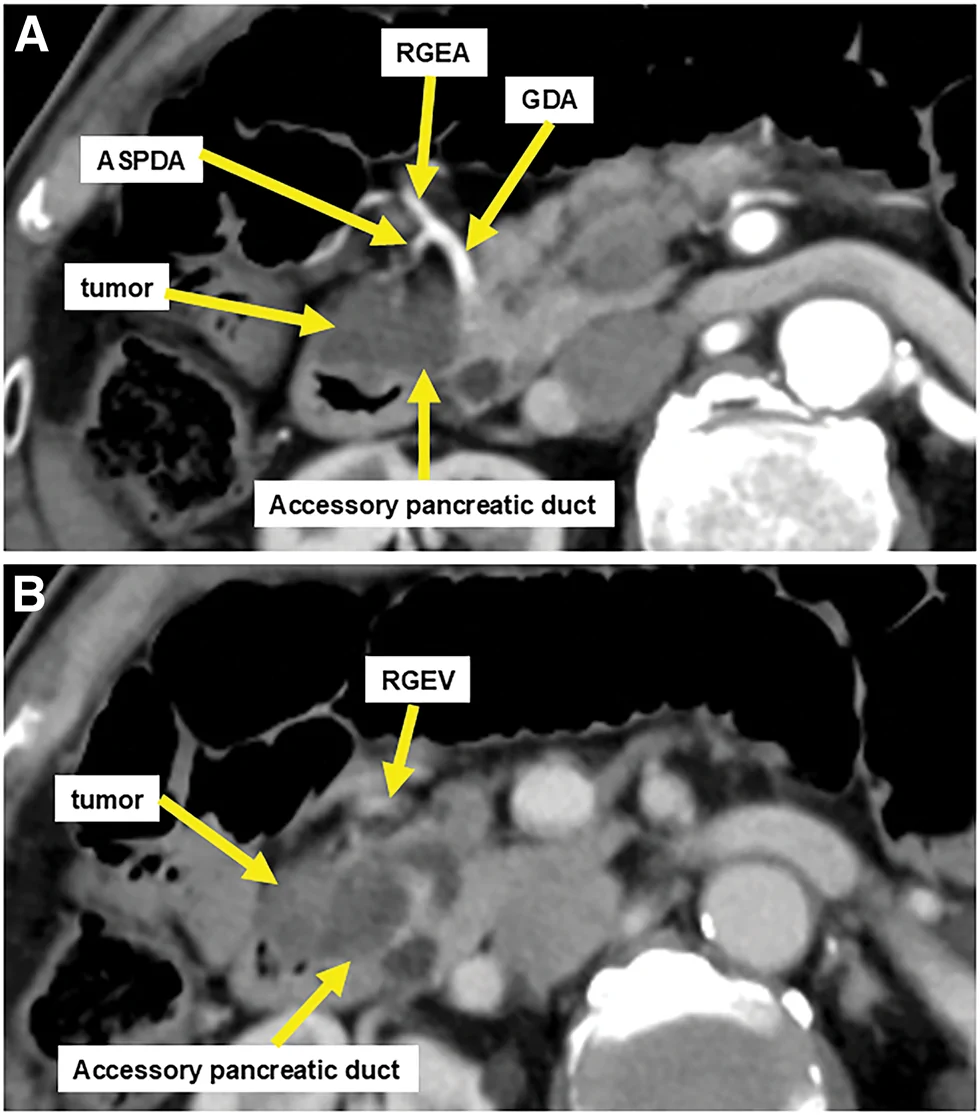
Preoperative CT findings. (A) CT revealed an approximately 25-mm tumor in the pancreatic head. The tumor was located approximately 11 mm from the GDA–RGEA axis. (B) The tumor was located approximately 13 mm from the RGEV. ASPDA, anterior superior pancreaticoduodenal artery; GDA, gastroduodenal artery; RGEA, right gastroepiploic artery; RGEV, right gastroepiploic vein
